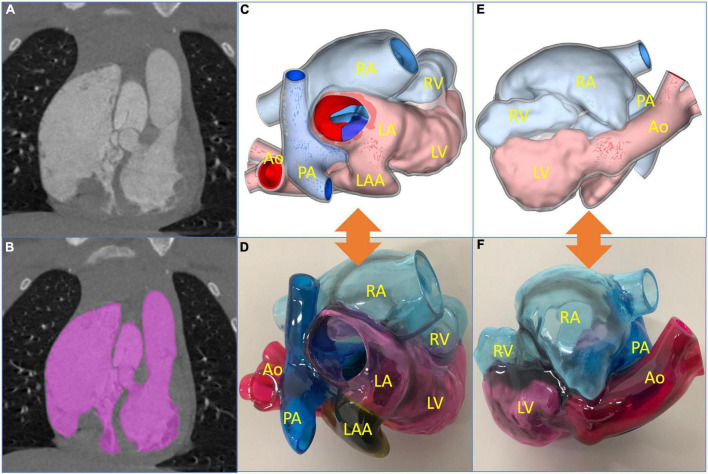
Reconstructed process of three-dimensional (3D) printed model and preoperative evaluation. (A) Original computed tomography angiography data. (B) Edition of the mask. (C,D) The 3D reconstructed and printed models are shown in the front view, respectively. (E,F) The 3D reconstructed and printed models are shown in the rearview, respectively. In the 3D printed model, the red part is the aorta (Ao), the blue part is the pulmonary artery (PA), the yellow part is the left atrial appendage (LAA), the pink part is the left atrium (LA) and left ventricle (LV), and the light blue part is right atrium (RA) and right ventricle (RV).
